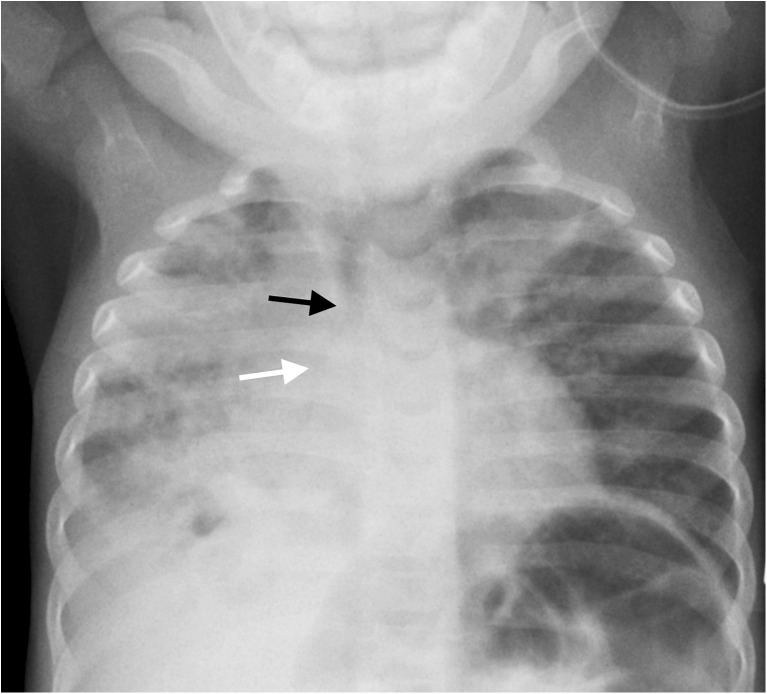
Anteroposterior chest radiograph requested in a malnourished 1-year-2-month-old boy presenting at a Médecins Sans Frontières site in the Central African Republic with an acute cough and lung crepitations. There was a specific request for the tele-reader to comment on any signs of pulmonary infection or signs of tuberculosis. The report read: “Infiltrates throughout the right lung and in the left upper lobe. Small cavity in the right lower lobe. Right hilar adenopathy narrows the right bronchus (*white arrow*) and mediastinal adenopathy slightly narrows the trachea (*black arrow*). Findings are highly indicative of primary TB”. Effusion on the right was not commented on
